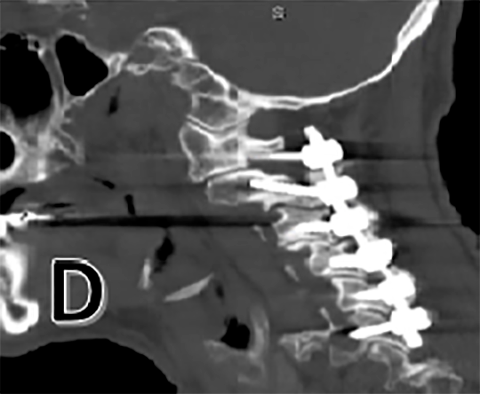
Post-operative sagittal CT scan Bone window showing C1-6 posterior cervical fusion.
